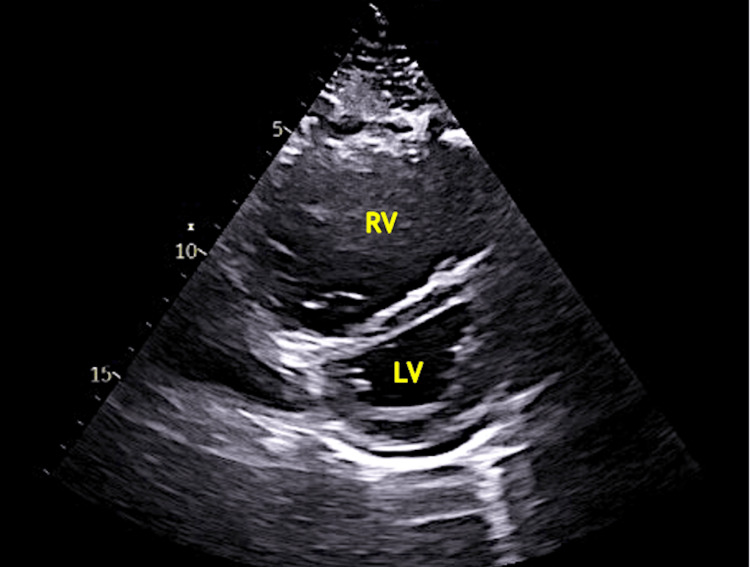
The parasternal short axis view demonstrates RV dilation with flattening of the interventricular septum due to increased RV pressures secondary to pulmonary hypertension RV - right ventricle; LV - left ventricle Original image by authors.
